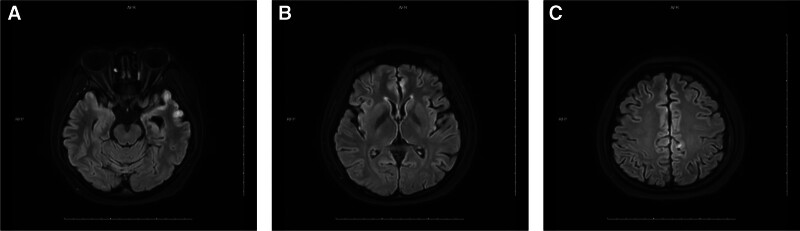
(A–C) March 1, 2024 – MRI demonstrates patchy T2/FLAIR hyperintensities in the left temporal and parietal lobes. MRI = magnetic resonance imaging.
